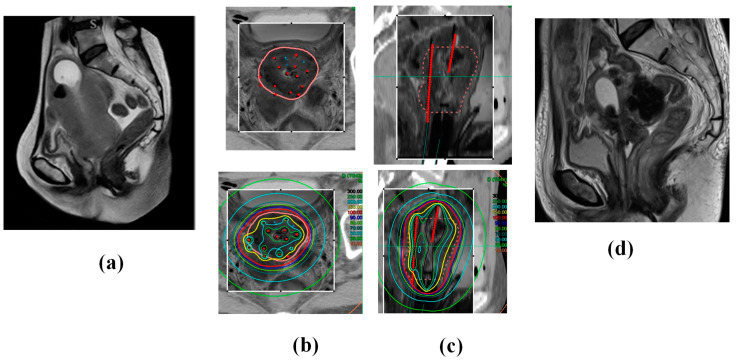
A 68-year-old woman presented with cervical cancer with uterine corpus invasion cT3bN1M0 (Stage 3B). The tumor exhibited significant extension into the lateral pelvic wall and corpus, with an initial gross tumor volume of 190.7 cc. The patient underwent whole-pelvic external beam radiotherapy (WP EBRT; 30 Gy in 15 fractions) with concurrent chemotherapy (40 mg/m^2^, q1 week CDDP × 5 times). The tumor volume reduced to 133.3 cc, and the patient underwent ISBT (30 Gy/5 fractions in 3 days administered twice daily). She continued to receive CS EBRT (10 Gy/10 fractions) and a lymph node boost EBRT (6 Gy/3 fractions). The patient remains in complete remission and alive 38 months post-treatment, with no evidence of recurrence. (a) Initial MRI image (Sagittal image). (b) Axial images and (c) Sagittal images of CT (outside of the rectangle) and MRI (inside of the rectangle) fusion images used in ISBT planning taken after the ISBT procedure. The pink line in the upper panel indicates the HR-CTV, and the red points and lines indicate the applicators. Note 100% isodose line (red line) fit to the HR-CTV in the bottom panel. (d) MRI image 4 months later (complete remission status).
